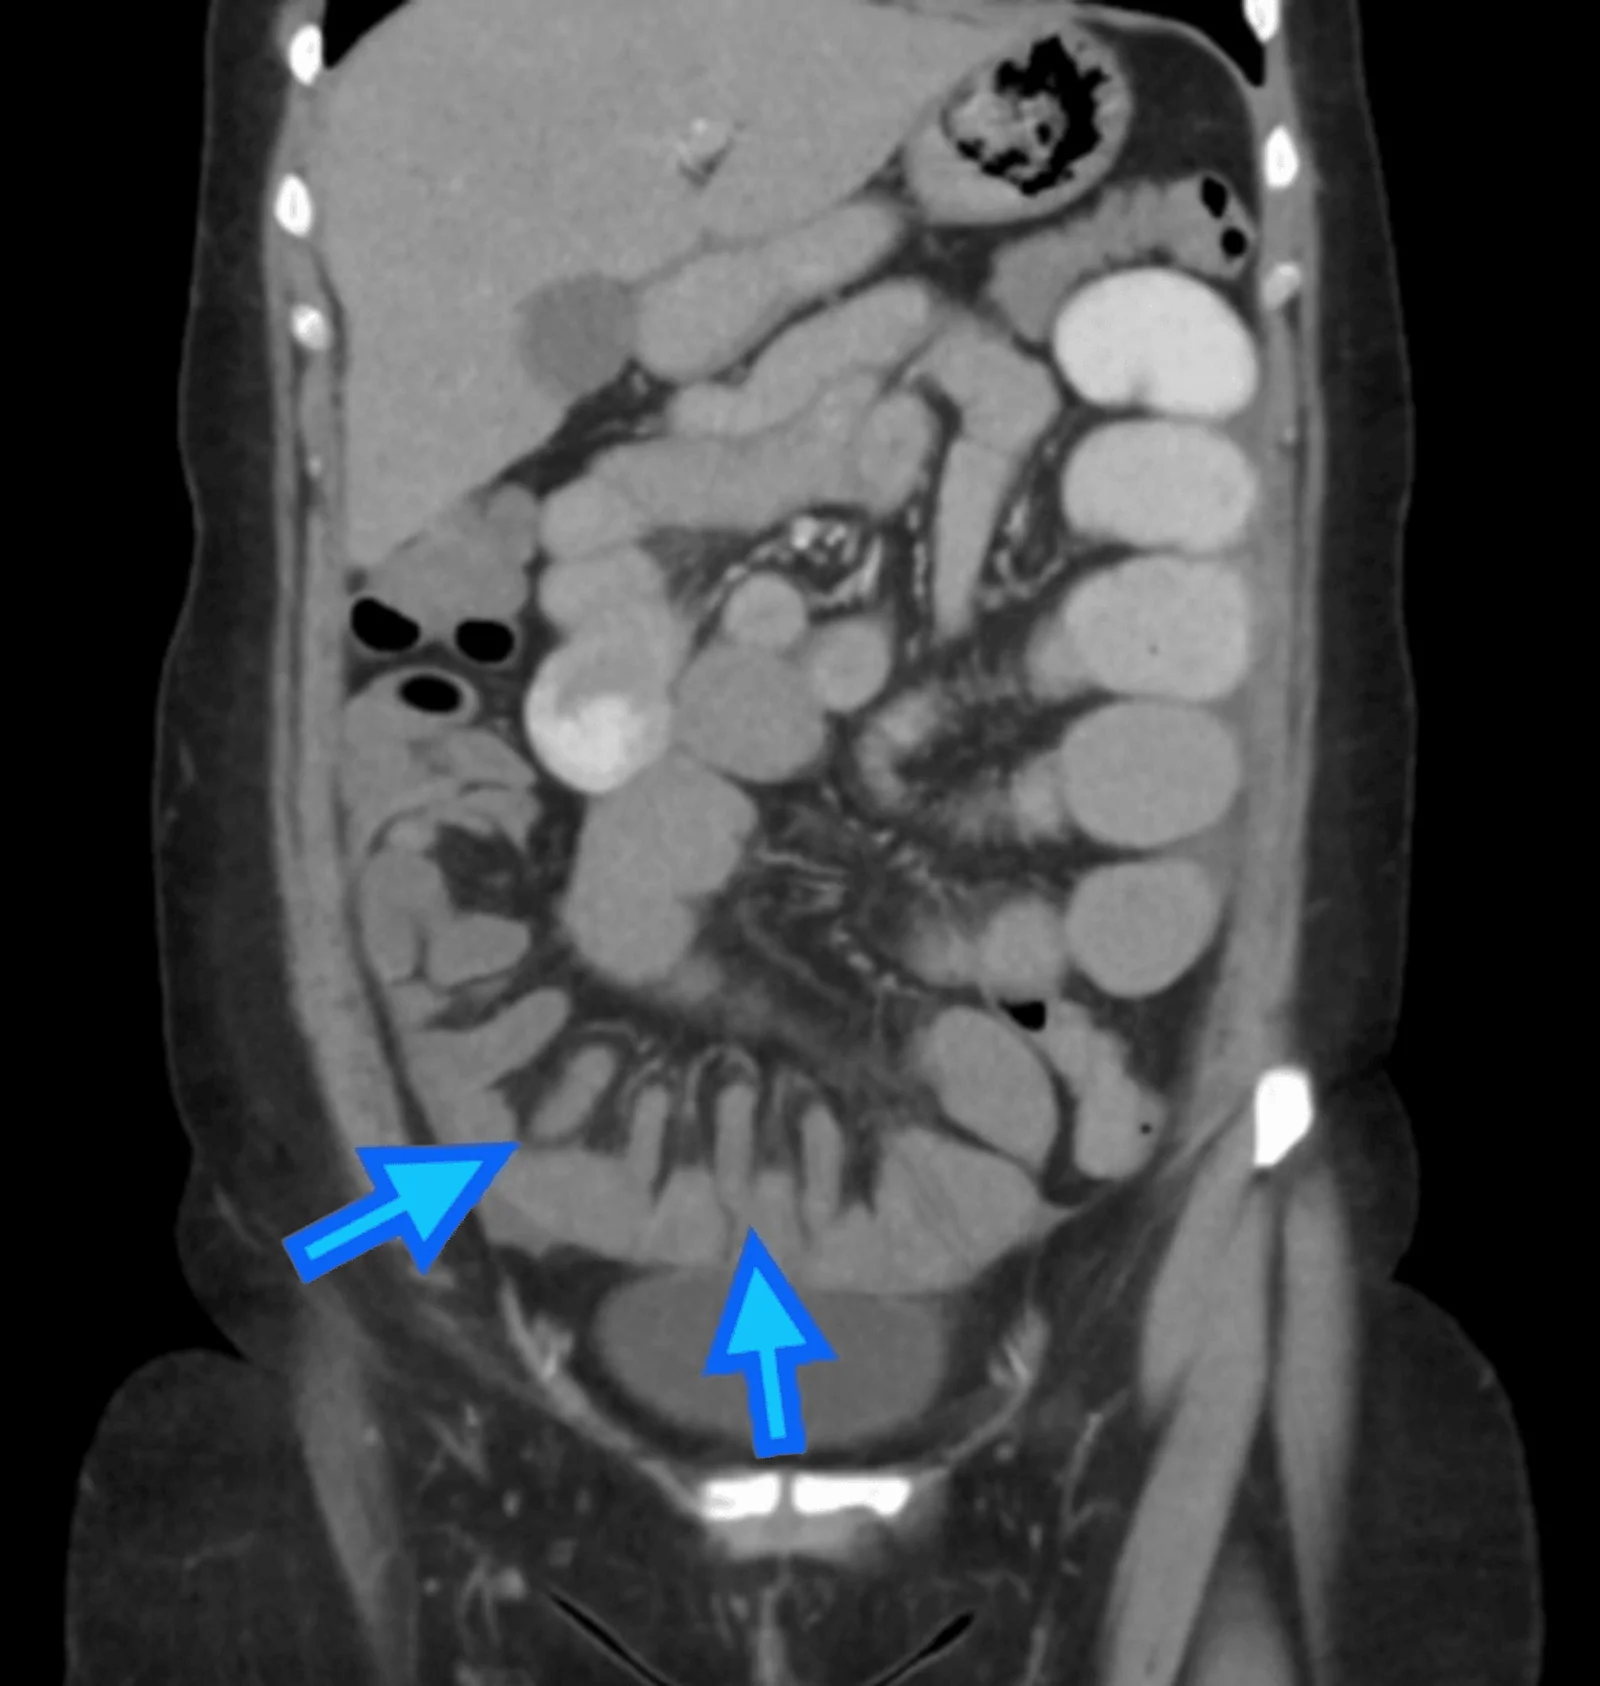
Coronal Contrast-Enhanced Abdominal CT Image A coronal view from the contrast-enhanced CT image demonstrating the verticalized, crowded, and U-shaped configuration of the small bowel loops trapped within the central abdomen, in a typical cocoon pattern (arrow), producing the characteristic “cauliflower sign.” CT: computed tomography
